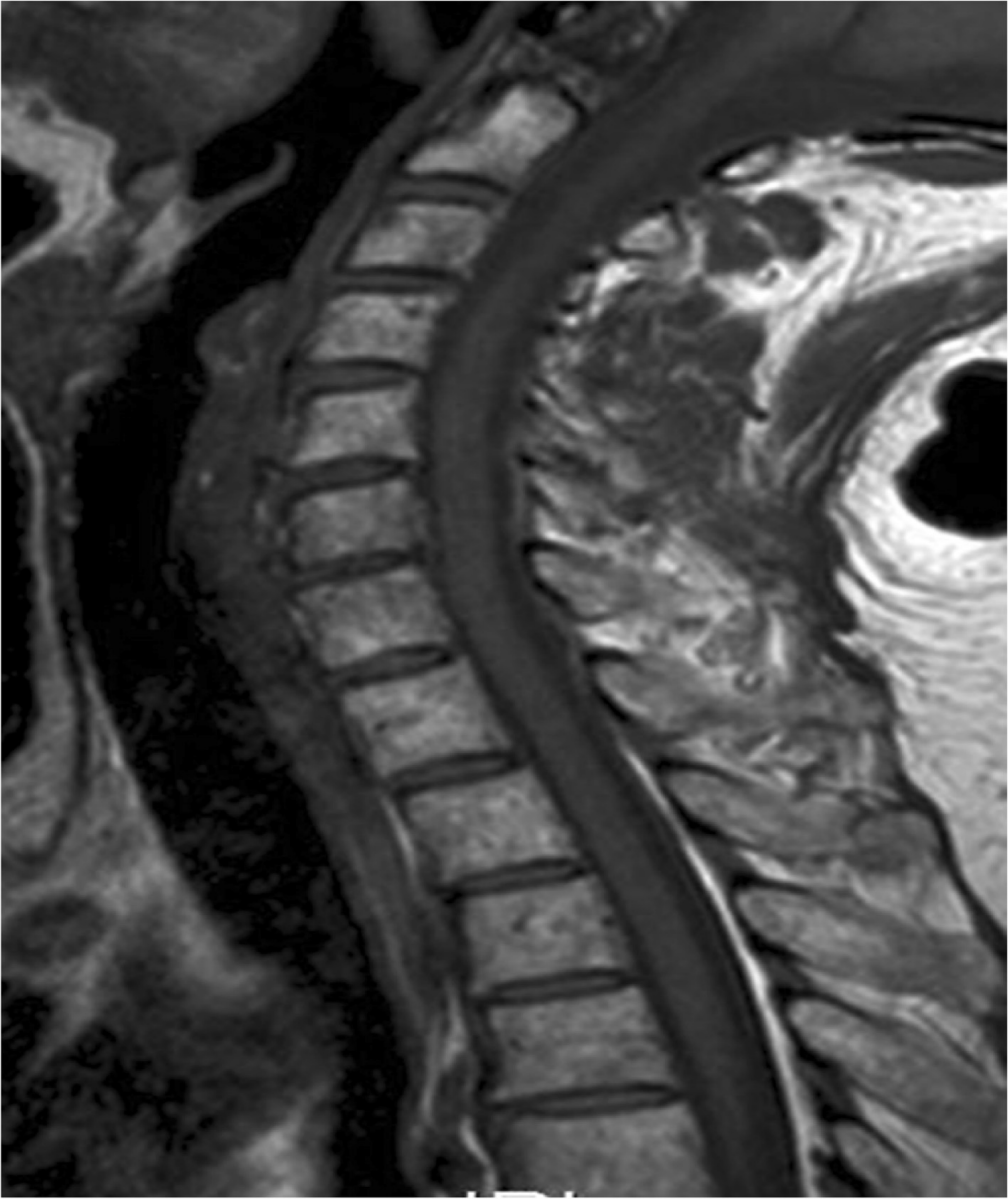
Cervical backbone magnetic resonance image after irradiation therapy and adjunctive chemotherapy. Her spinal cord compression is shown to have disappeared and the intrapharengeal lumen is free of plasmacytoma.
